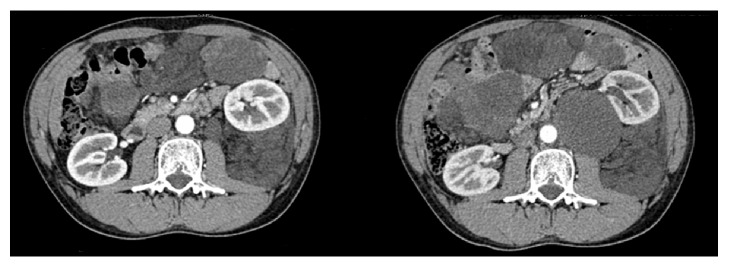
Multidetector computed tomography (MDCT) scan after contrast medium (arterial phase). Progression of abdominal metastasis from a myxoid liposarcoma after two cycles of high-dose prolonged-infusion ifosfamide.
